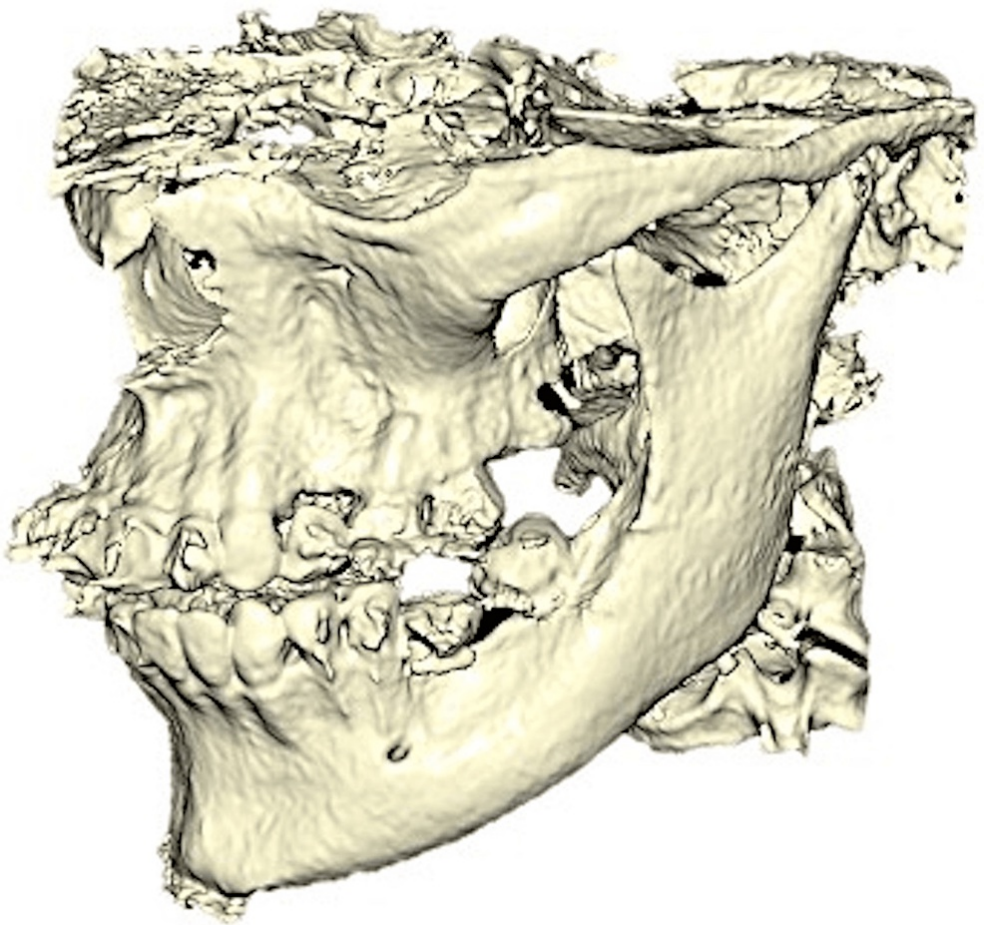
Mandibular jaw (cone beam CT) image was converted into an STL file.
